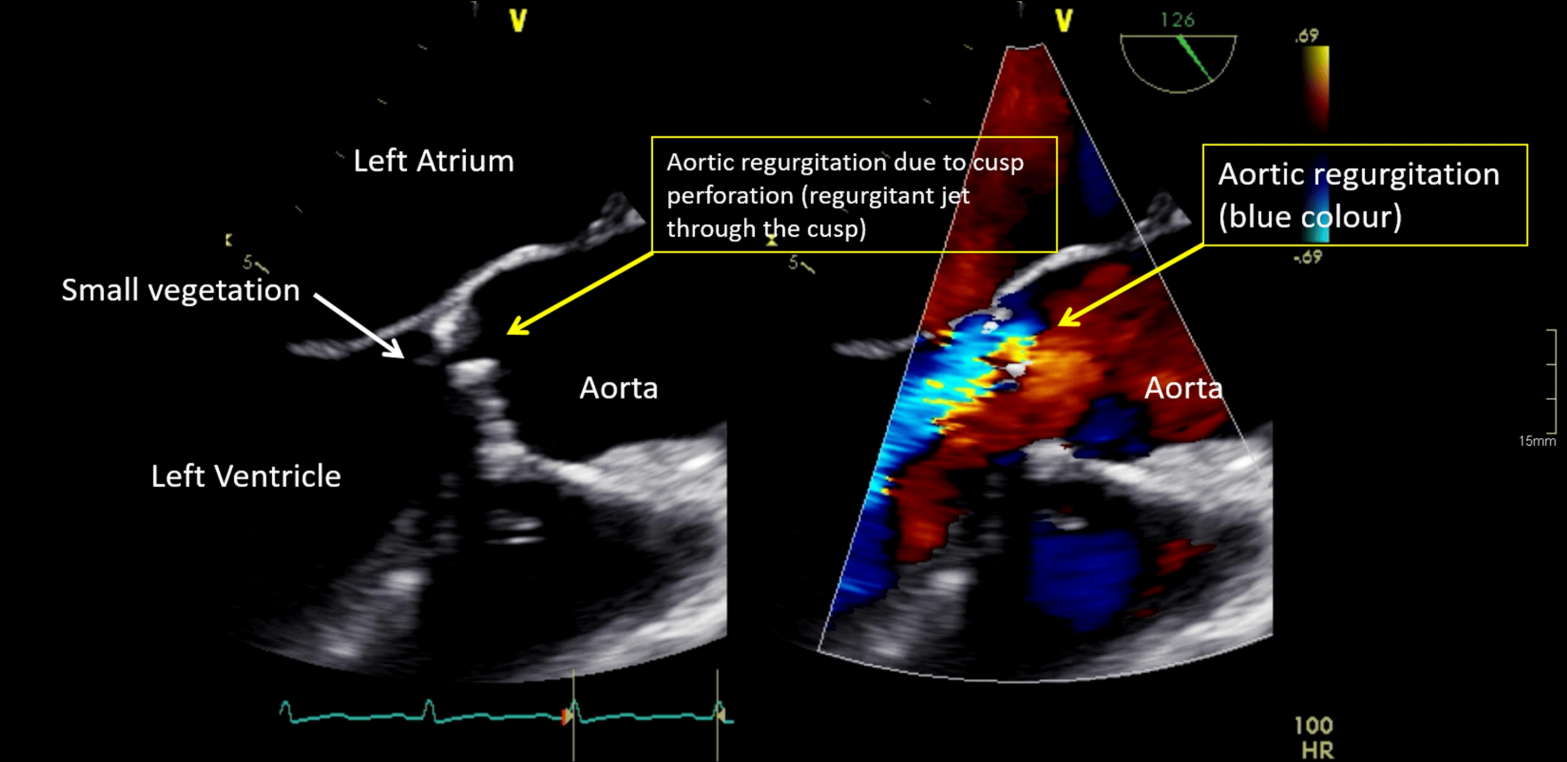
Transesophageal echocardiogram performed on day 11.
